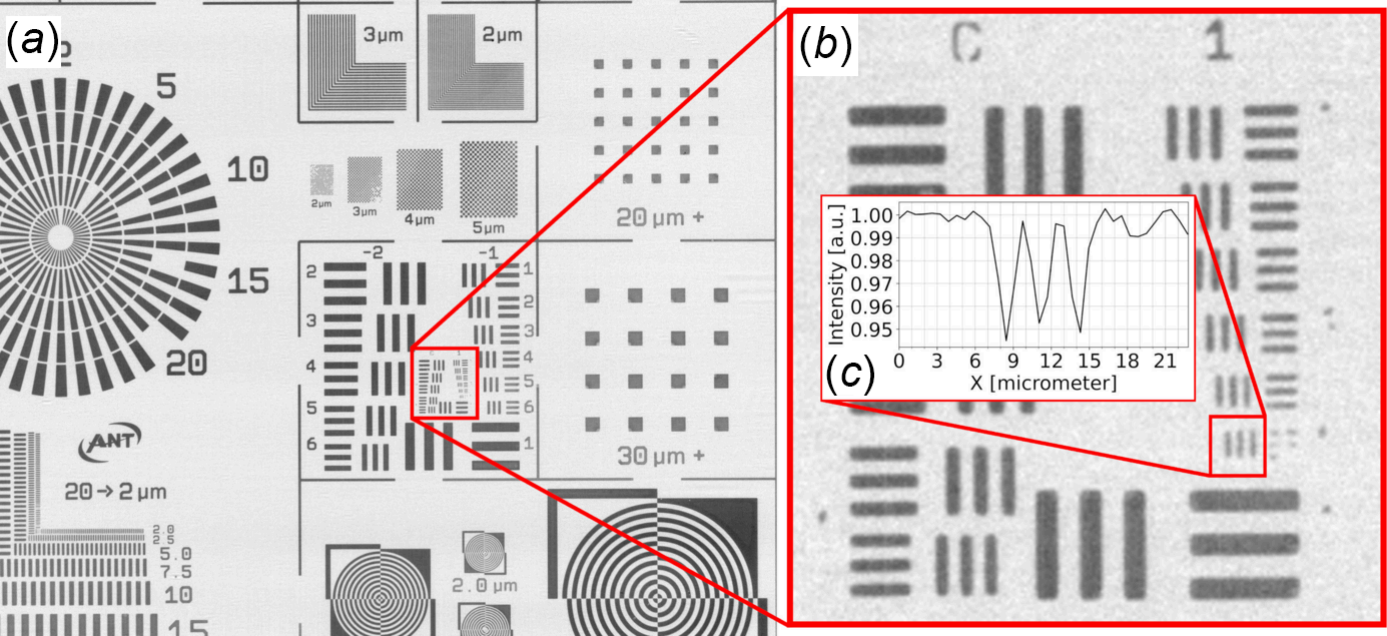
(*a*) Radiograph of the X-ray resolution target (XRCAL-2µm, Applied Nanotools Inc., Canada). (*b*) Detail (enlarged) of (*a*) showing series 0 and 1 of a micro-USAF test pattern. Element 6 of series 1 of the pattern (contoured in red) corresponds to line pairs of 0.357 lp µm^−1^. (*c*) Intensity horizontal line profile through (*b*).
